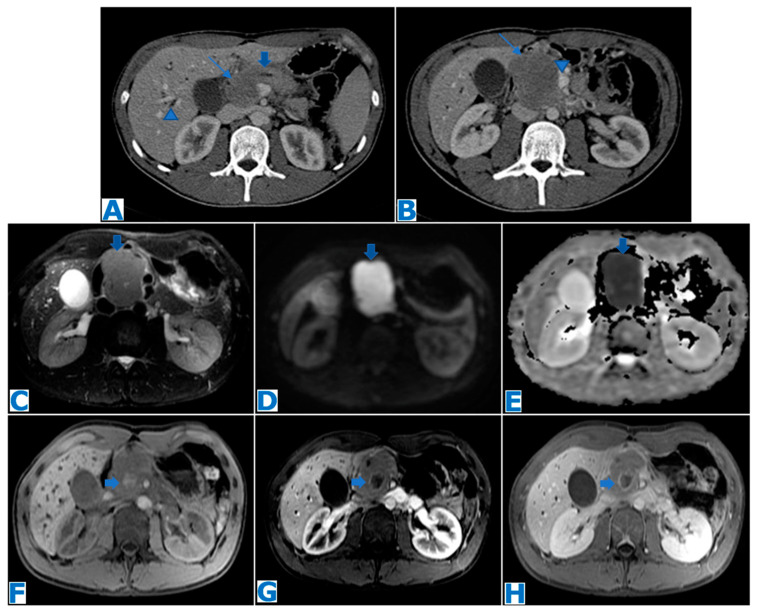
(A,B): axial CE portal venous phase CT. (C): axial FST2WI, (D): DWI, (E): ADC, (F): axial NCE FST1WI, (G): axial CE pancreatic parenchymal phase FST1W1, (H): axial CE portal venous phase FST1W1. Primary pancreatic lymphoma (focal form) in a 26-year-old patient with obstructive jaundice. CT revealed a hypovascular mass in the head of the pancreas (thin arrows in (A,B)), with minimal bile (arrowhead in (A)) and MPD dilatation (thick arrow in (A)), no distal parenchymal atrophy and abutment of the superior mesenteric vein (arrowhead in (B)). Note in the T2WI a homogeneous slightly hyperintense mass (arrow in (C)) with marked diffusion restriction (arrows in (D,E)) and its hypovascularity following intravenous contrast administration. Considering the tumour size, its homogeneity, marked diffusion restriction, growth pattern and hypovascularity with minimal MPD and biliary dilatation, lymphoma was one of the top possibilities on the differential diagnosis list. Note a small haematoma in the center of the mass (arrows on (F–H)) secondary to a EUS-guided biopsy, which concluded Burkitt lymphoma. The patient was successfully treated with chemotherapy, obtaining a complete remission.
